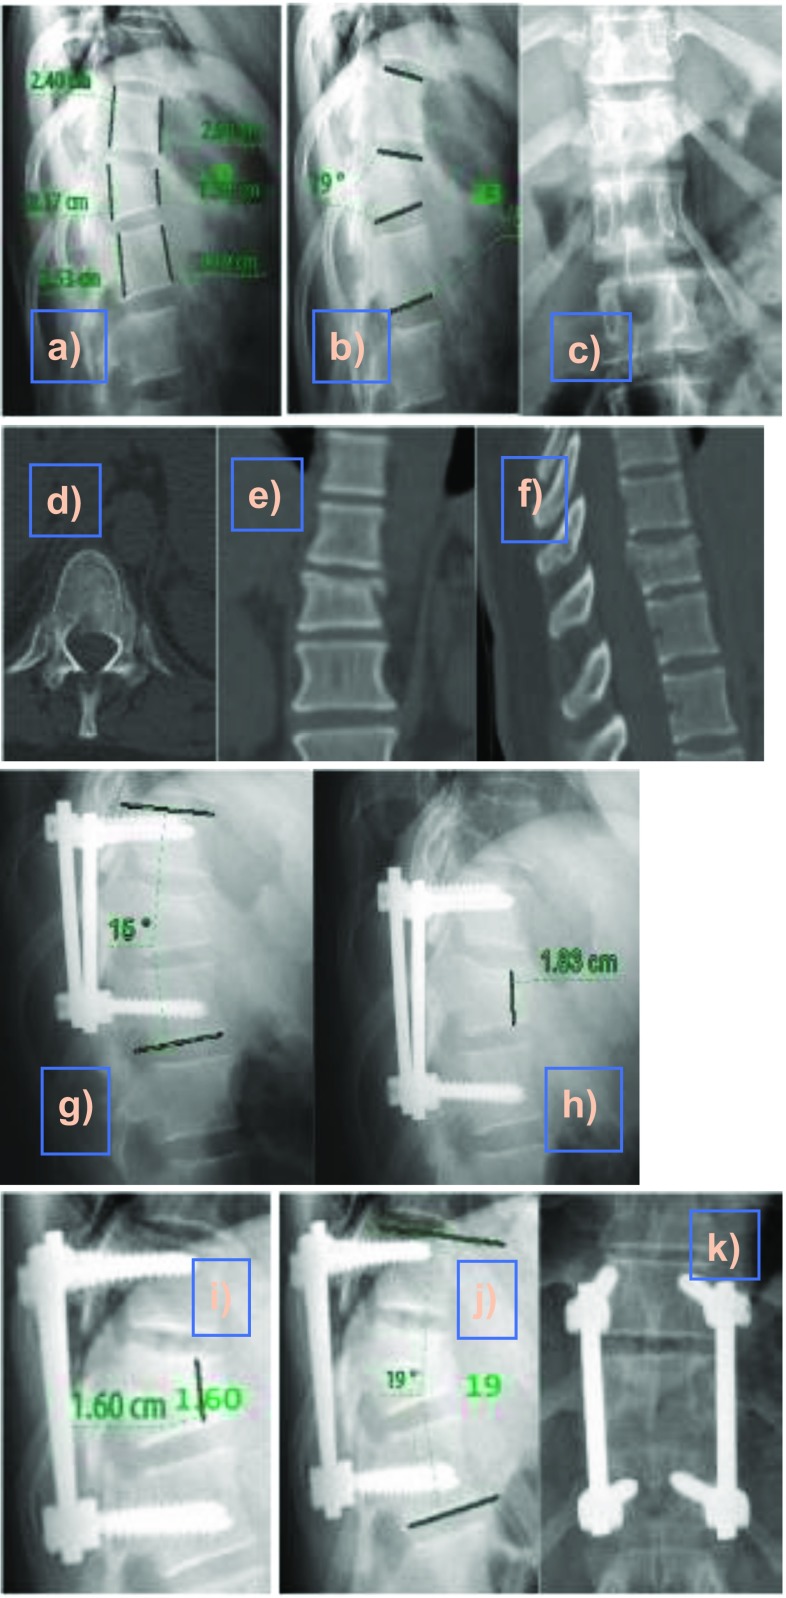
D11 fracture after fall from a height in a 35-year-old male without neurological deficit. (a–c) Preoperative measurements of angular kyphosis and anterior vertebral height; (d–f) preoperative CT; (g, h) immediately post-operative lateral X-rays after application of a Legacy System, and (i–k) follow-up AP and lateral X-rays at 12 months.
